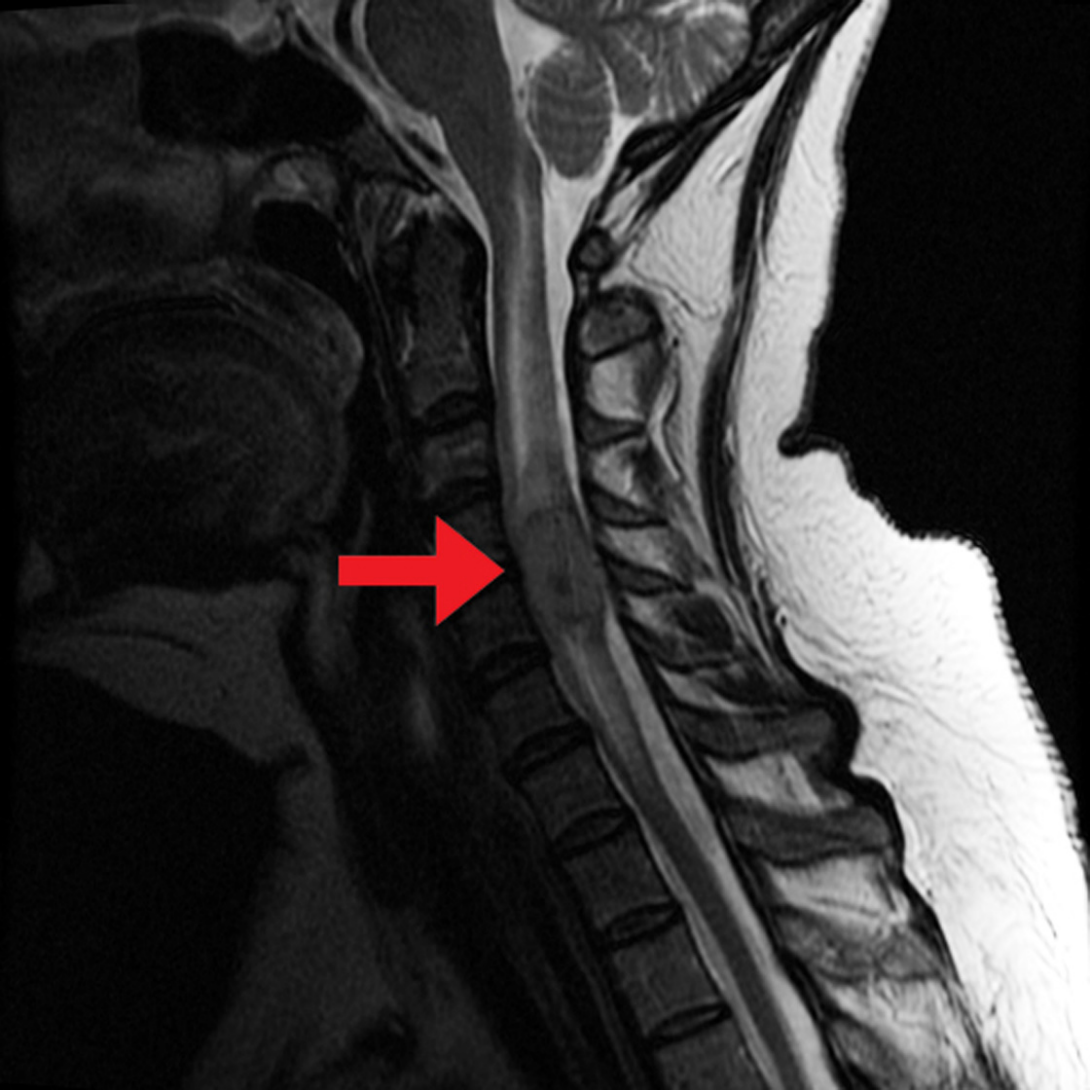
T2‐Weighted sequence of MRI of the cervical spine in sagittal view, showing a 2.1*1.3 centimeter heterogeneous enhancing solid intramedullary mass involving the cervical spine at C4‐C5 level with expansion of the cord.
